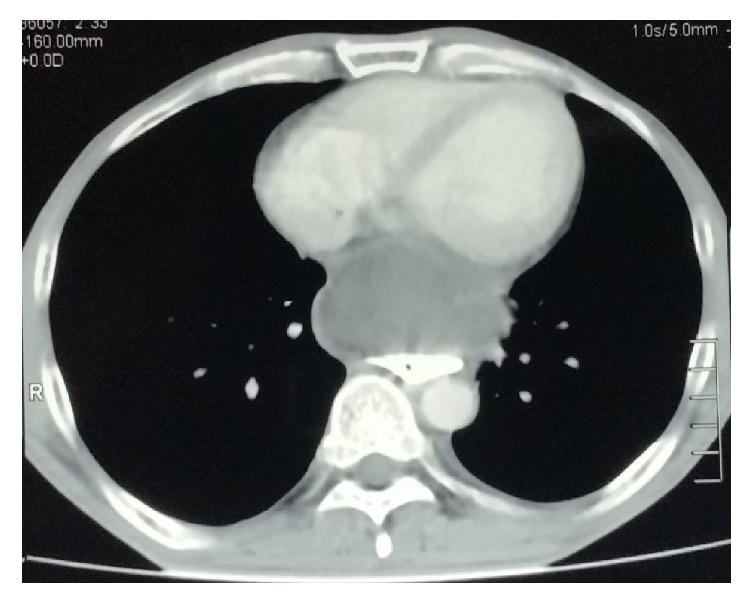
CT chest showing mediastinal pseudocyst compressing the esophagus.
